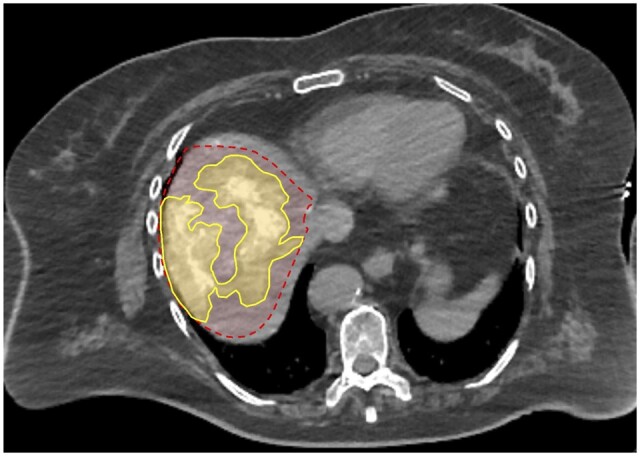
Large areas of hypovascularity within the tumour were major contributors to overestimated volume measurements by the Couinaud anatomic model. Shown is a 7.9-cm cholangiocarcinoma tumour of the dome of segment 8. Its corresponding tumoural angiosome, as seen on Angio-CT (yellow area; Patient 22 from Supplementary Table S1), encompasses only a portion of the true tumour volume. The lateral aspect of the tumoural angiosome was supplied by an arterial branch to segment 8, whilst the medial aspect was supplied by an arterial branch of segment 4. The corresponding Couinaud segment is shown in red.
